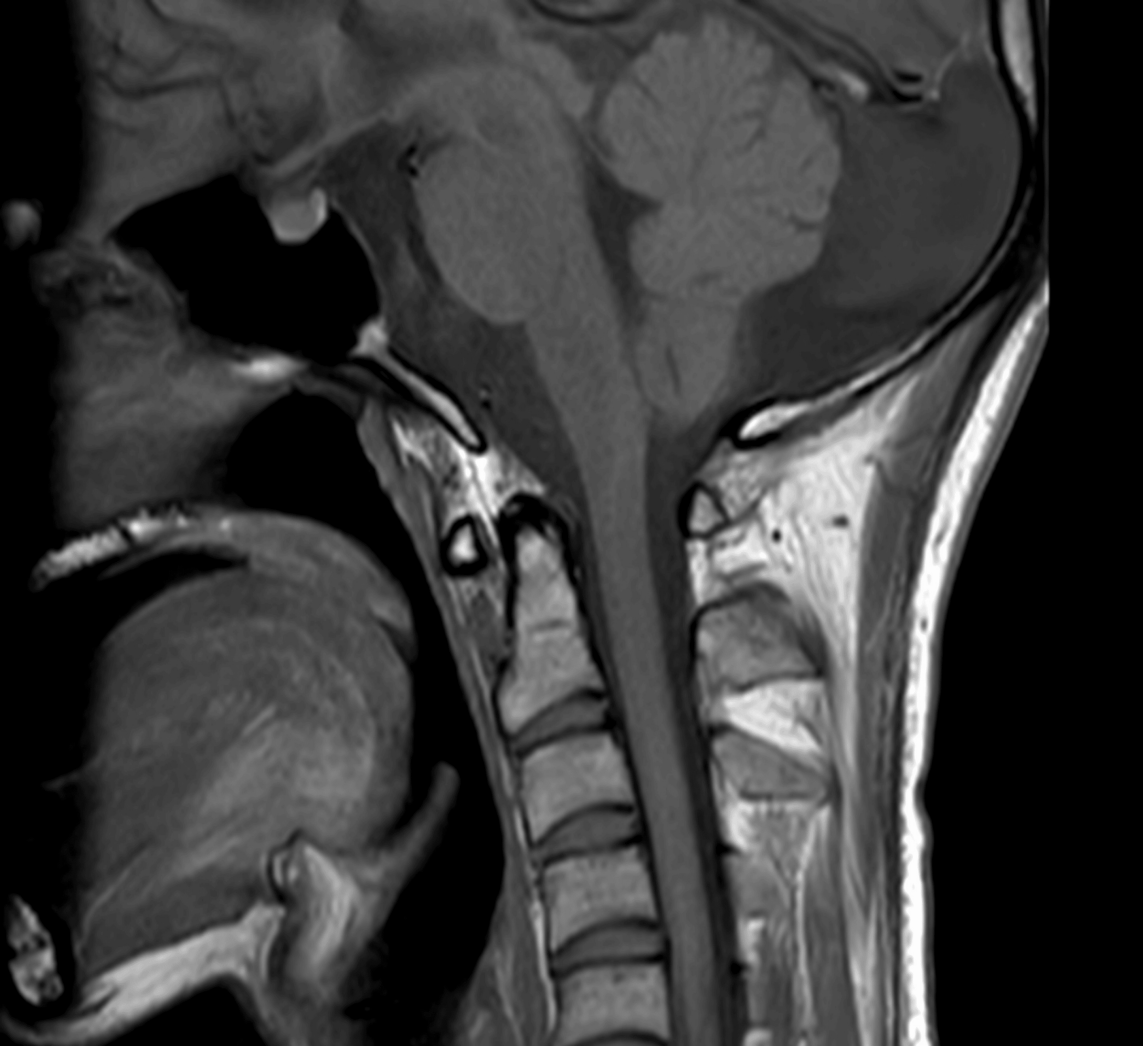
Postoperative follow-up image. T1-weighted sagittal images obtained two months postoperatively showed no evidence of residual tumor or recurrence.
